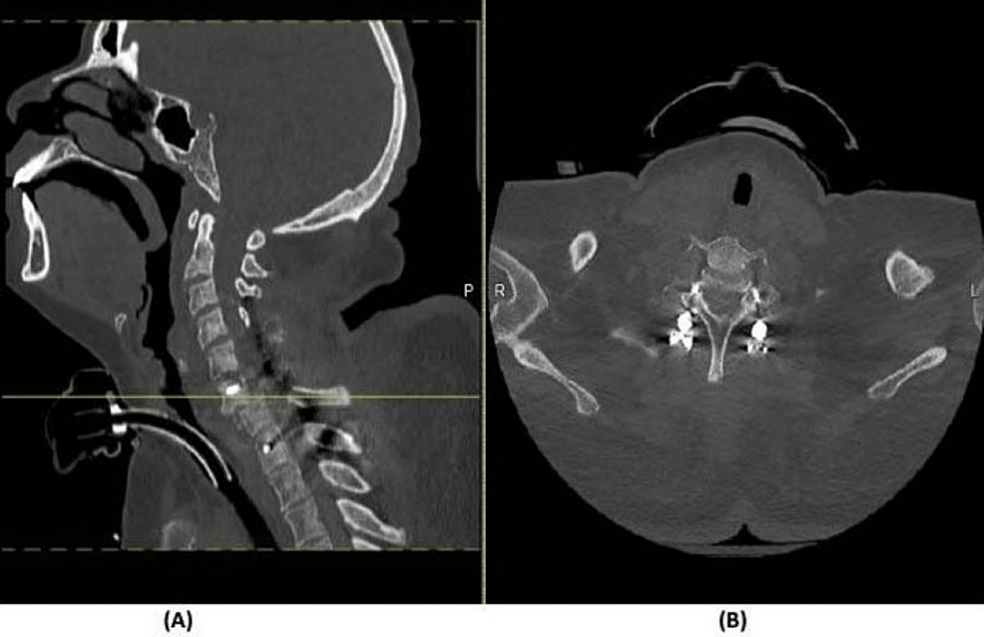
CT scan of the final C6-7 fracture reduction (A) Sagittal spine CT demonstrating near total anatomical reduction of C6–C7 fracture dislocation; (B) axial spine CT of the corresponding level of C6–C7 vertebral body.
